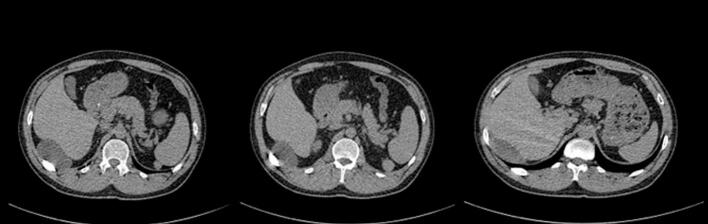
Abdominal CT scan W/O contrast was done which a cystic lesion with the size of 68*52*48 in the right sub diaphragm.
